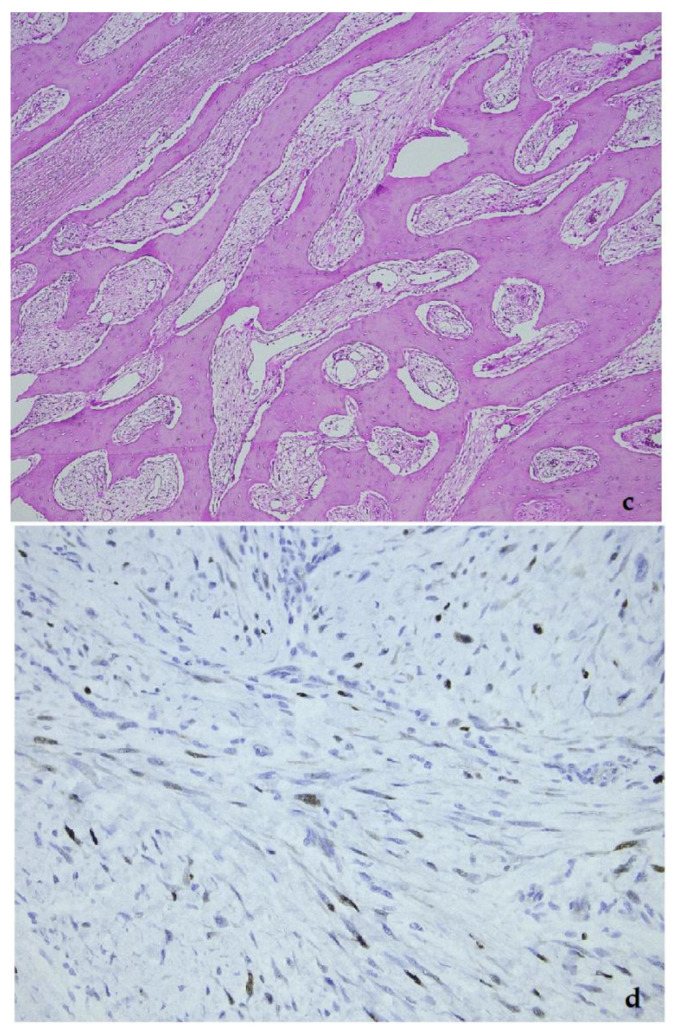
Parosteal osteosarcoma, typically presenting as a mineralized mass at the back of the distal femur, X-ray (a), and whole mount section (b). At high power, the tumor consists of parallel bone trabeculae and a typical spindle cell proliferation (c). *MDM2* expression is present as well (d).
